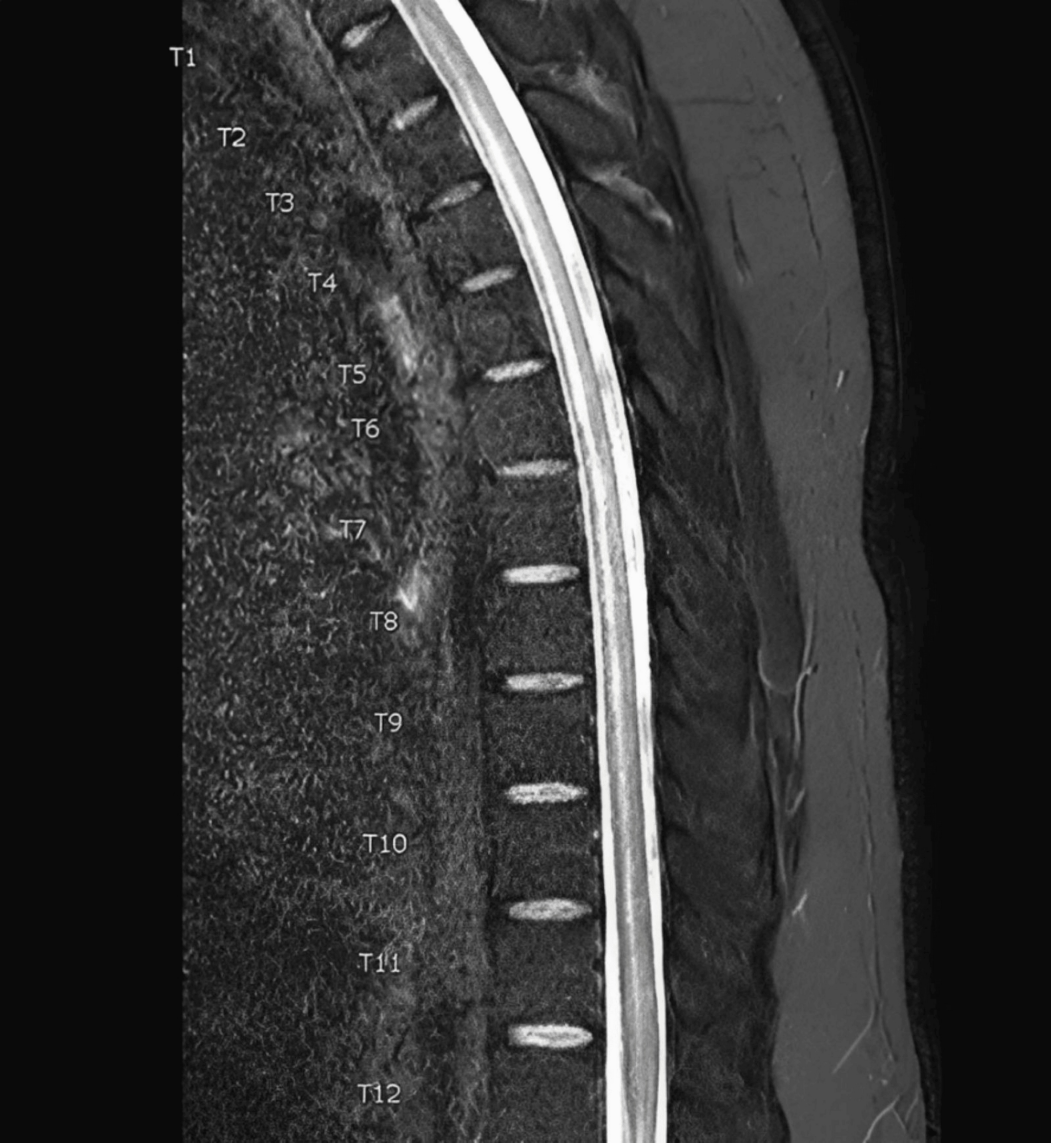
MRI of the thoracic spine Sagittal image demonstrating a long segment of hyperintensity within the spinal cord parenchyma, extending from T1 to T10 (arrows), consistent with LETM. LETM, longitudinally extensive transverse myelitis
